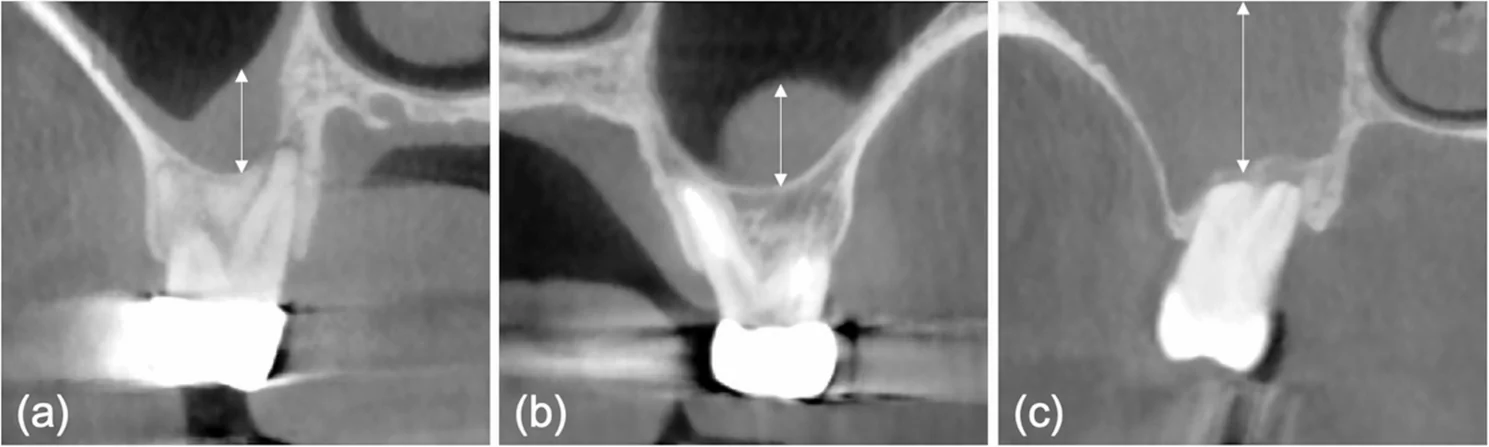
Radiographic assessment of maxillary sinus membrane thickening (SMT). a Sagittal CBCT view showing a flat SMT pattern (> 2 mm). b Polypoid morphology characterized by a dome-shaped lesion. c Mucocele-like appearance with complete sinus opacification. The measurement representsthe maximum thickness perpendicular to the bony wall
